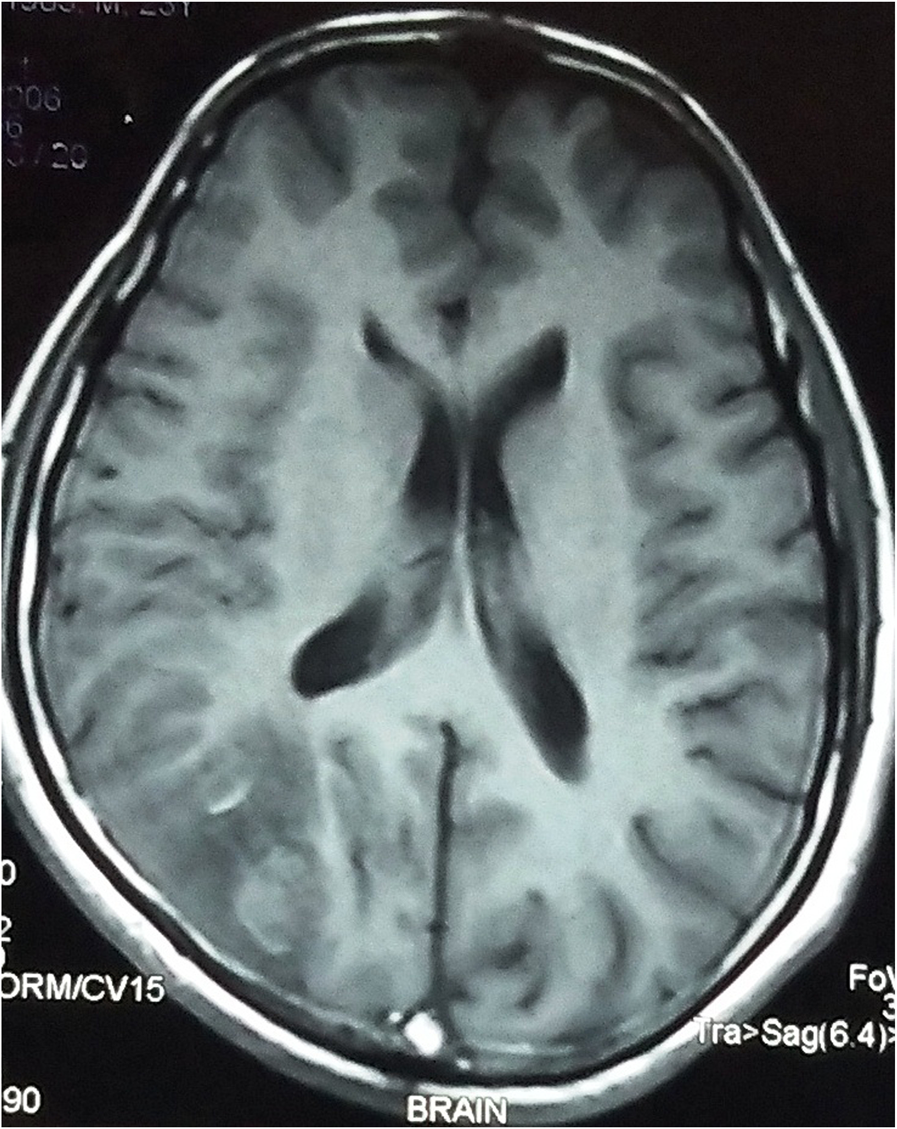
T1-weighted axial images at the same levels show the areas of hyperintensity in the above lesion due to hemorrhage. The normal flow void in the posterior part of superior sagittal sinus is replaced by a hyperintense signal on both T1-weighted and T2-weighted images due to thrombosis
